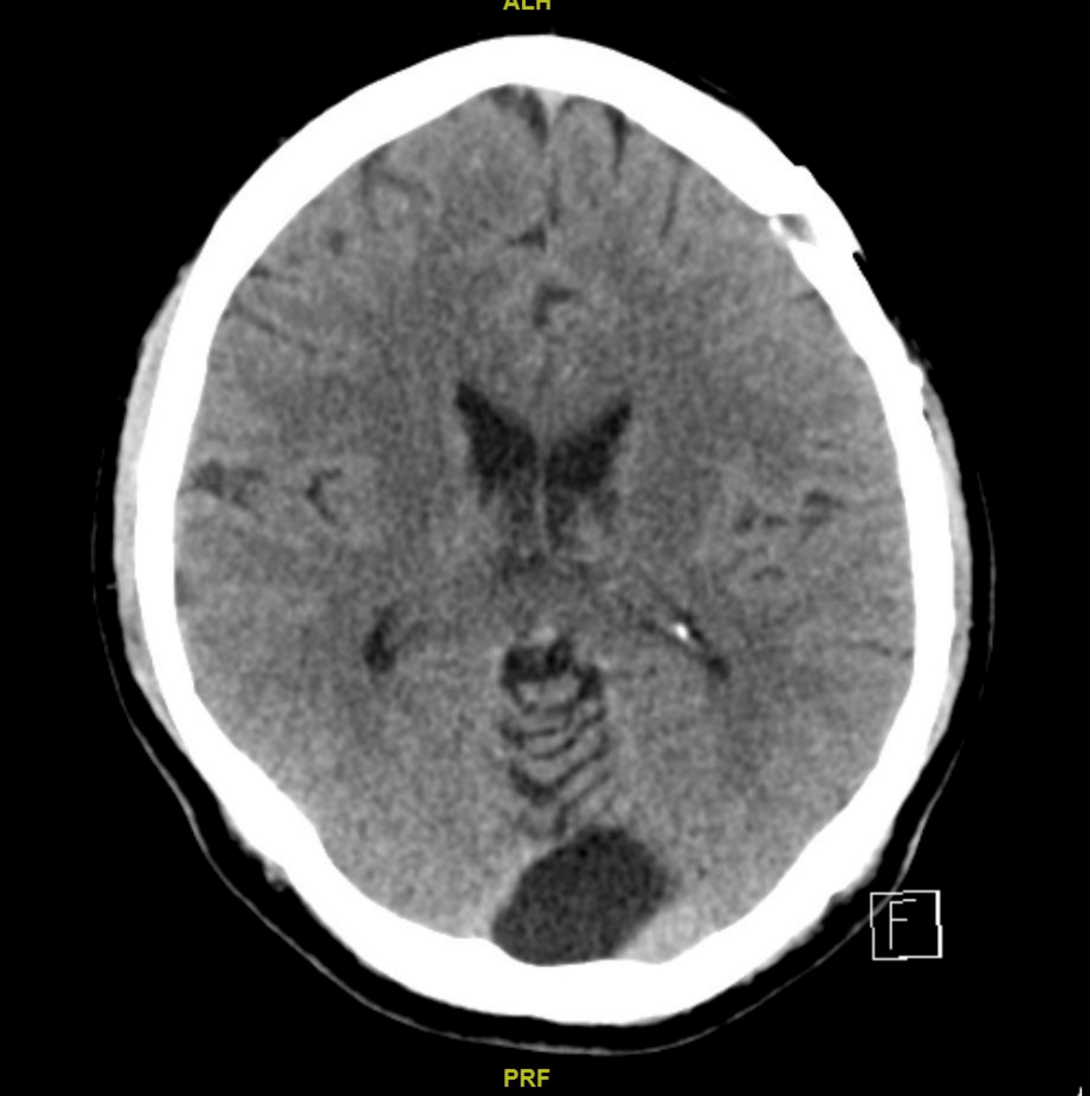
CT of the brain nine months after discharge showing complete resolution of bilateral subdural hematoma.
